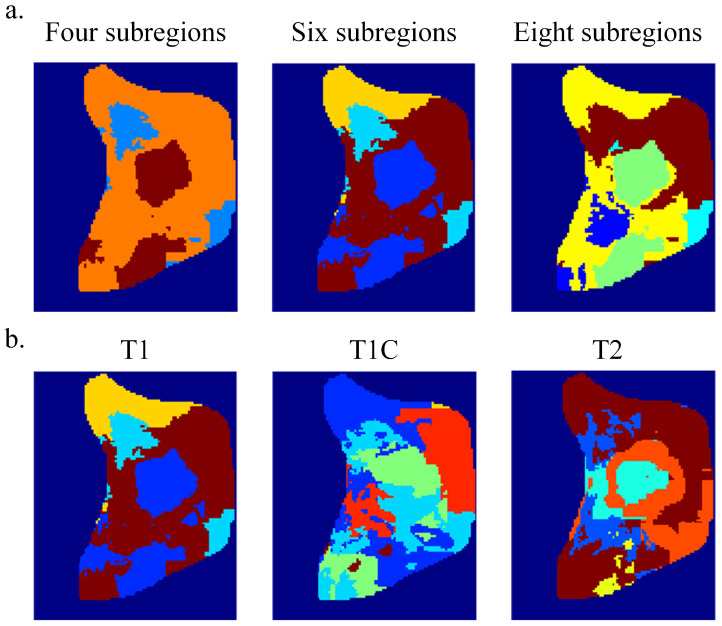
Habitat imaging results: (a) Comparison of habitat partitioning under different clustering numbers (k=4,6, and 8). (b) Representative habitat maps derived from T1, T1C, and T2 MRI sequences using the optimal clustering number (k=6). Different colors represent distinct habitat subregions identified by clustering.
